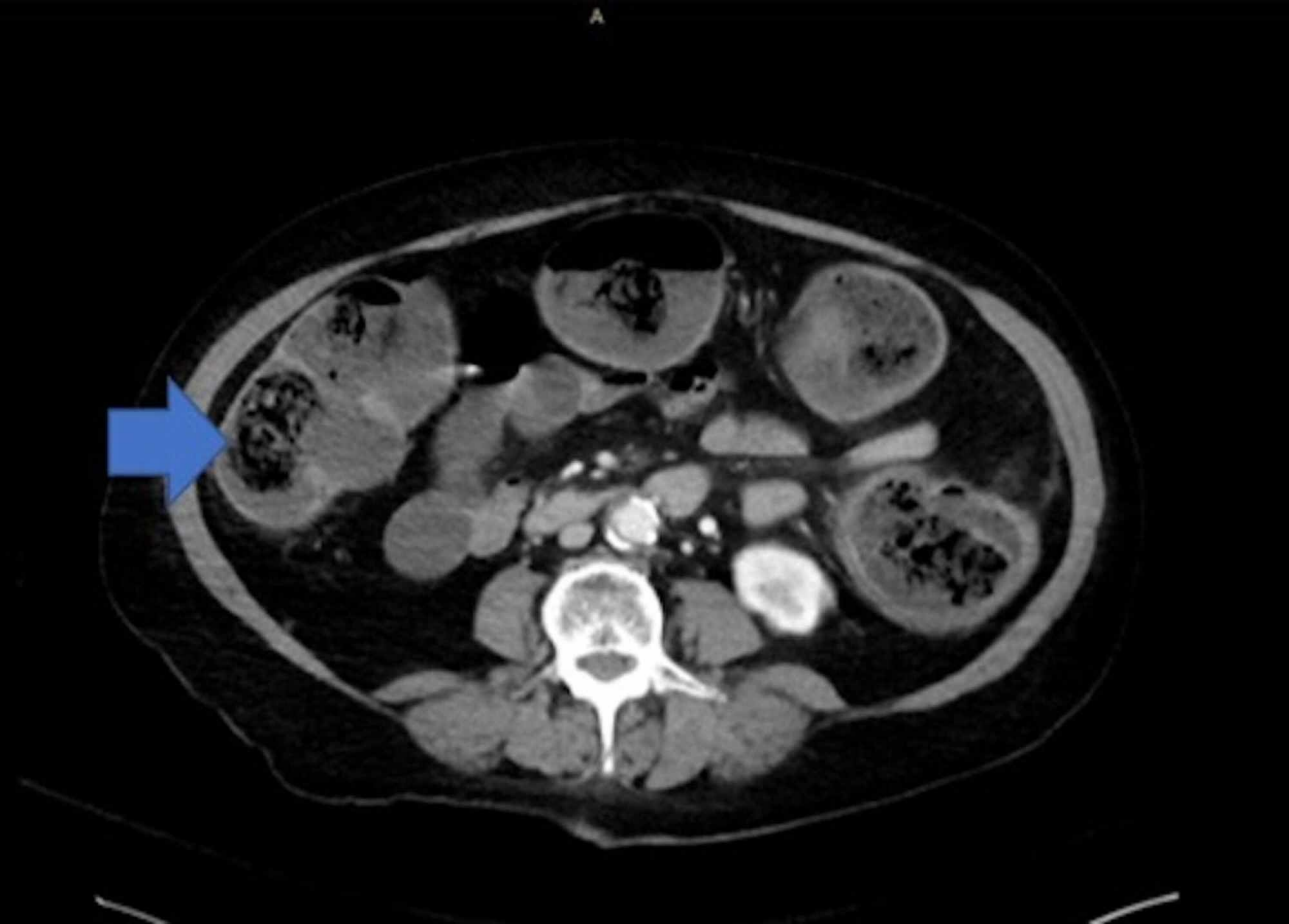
CT scan showing loaded colon with fecal matter CT, computed tomography
